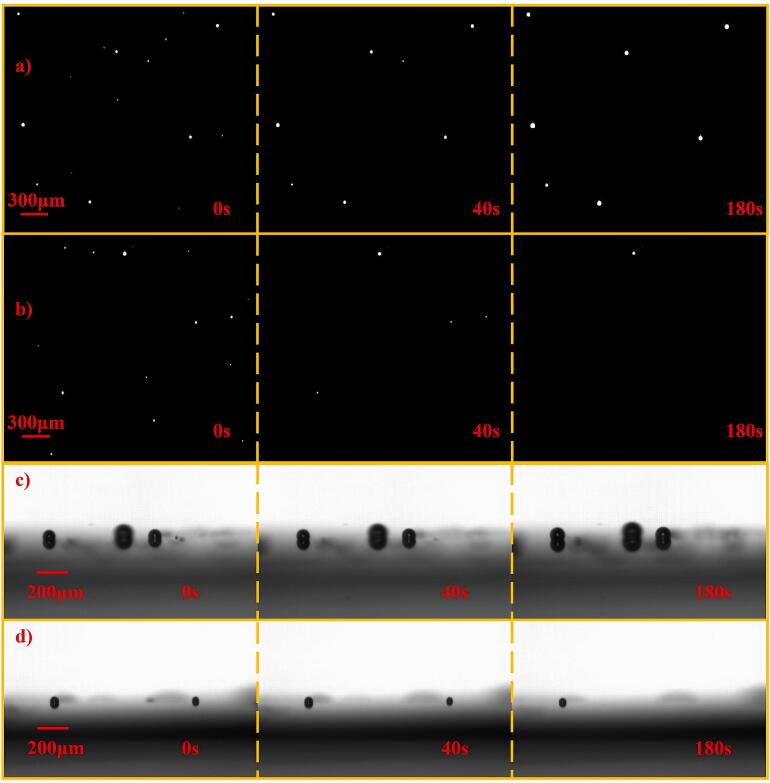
Top-view images of surface microbubbles on a hydrophobic surface in OW (a) and SW (b), and side-view images in OW (c) and SW (d), acquired at 0 s, 40 s, and 180 s after ultrasonication.
